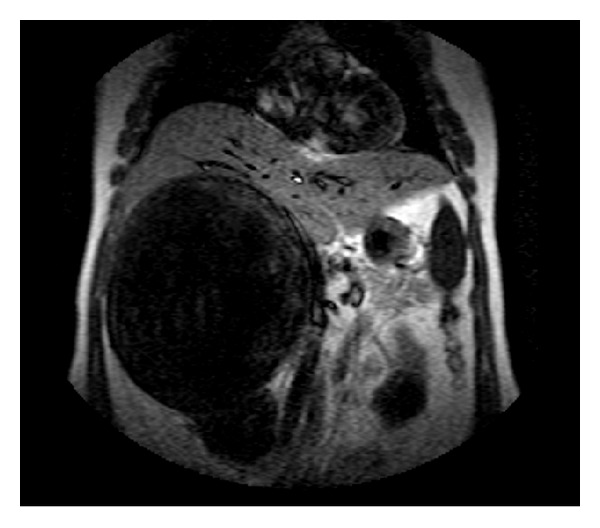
Coronal plane of patients preoperative MRI scan, demonstrating the right quadrant huge mass.
